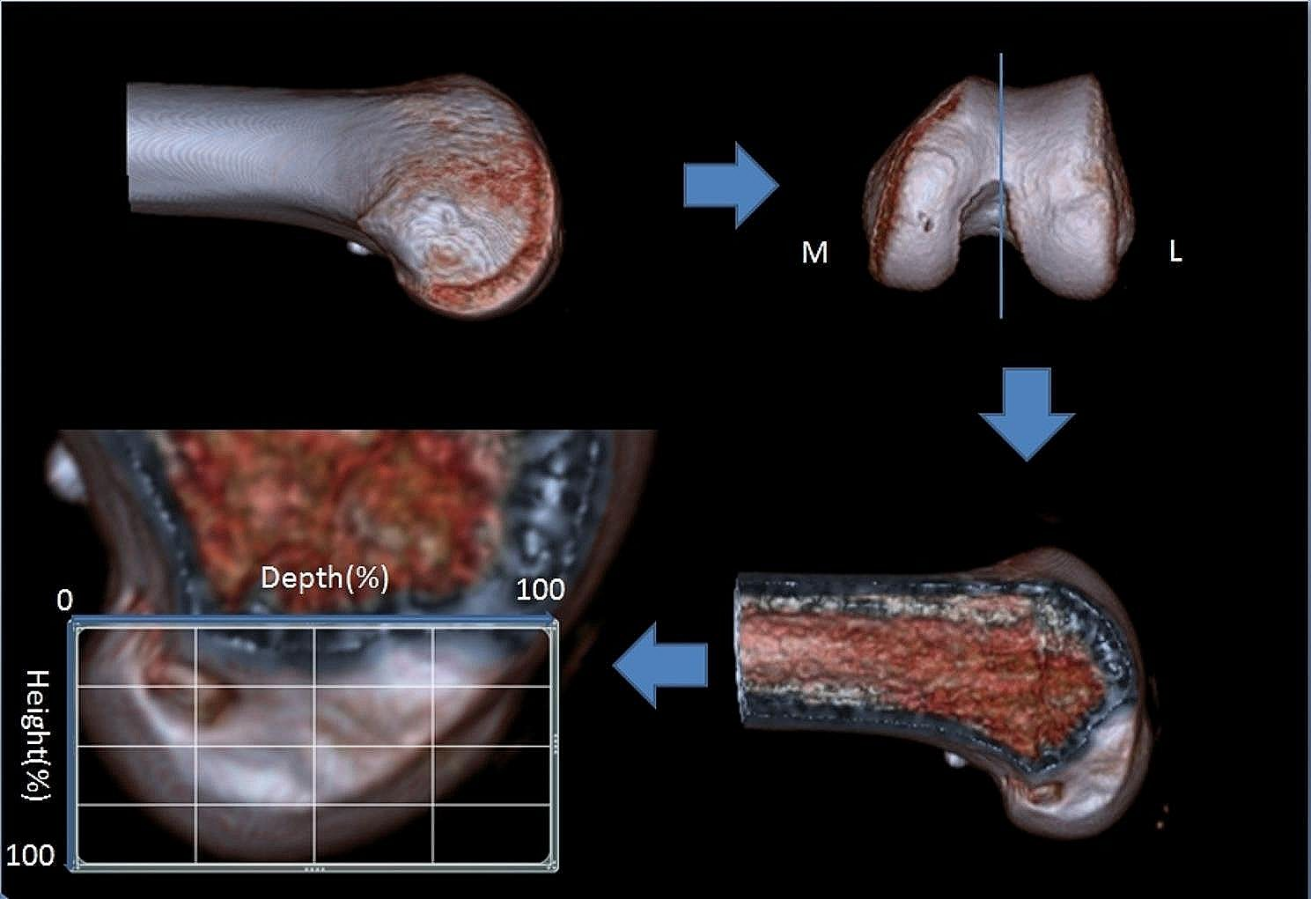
The M–L view of thelateral femoral condyle was obtained from the 3D CT of the distal femur in the strictly lateral position, where both condyles were superimposed. 3D CT of the medial–lateral (M–L) view was applied to 4 by 4 grid based on the quadrant method. M–L, medial–lateral
